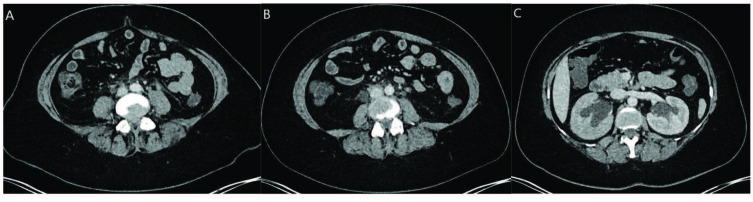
A and B, retroperitoneal fibrosis. Contrast-enhanced axial images (A) and coronal image (B) of computed tomography of the abdomen show heterogenous soft tissue densities surrounding aorta in the retroperitoneum at the infrarenal level. Soft tissue densities severely surround aorta, aortic bifurcation and main iliac arteries bilaterally (black arrow). (Image courtesy of Emetullah CİNDİL).
